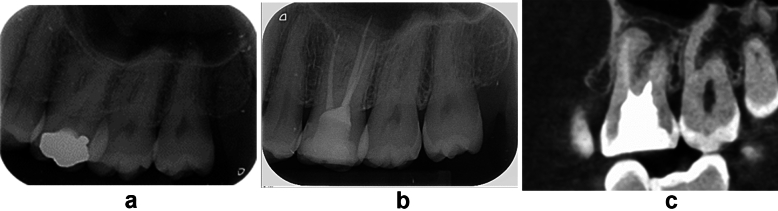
(a) Abnormal anatomy found in mesiobuccal root of #26 with a periapical lesion, which was hidden on the radiograph by the Mesiobuccal root, (b) tooth obturated (c) CBCT scan at one-year recall visit.
